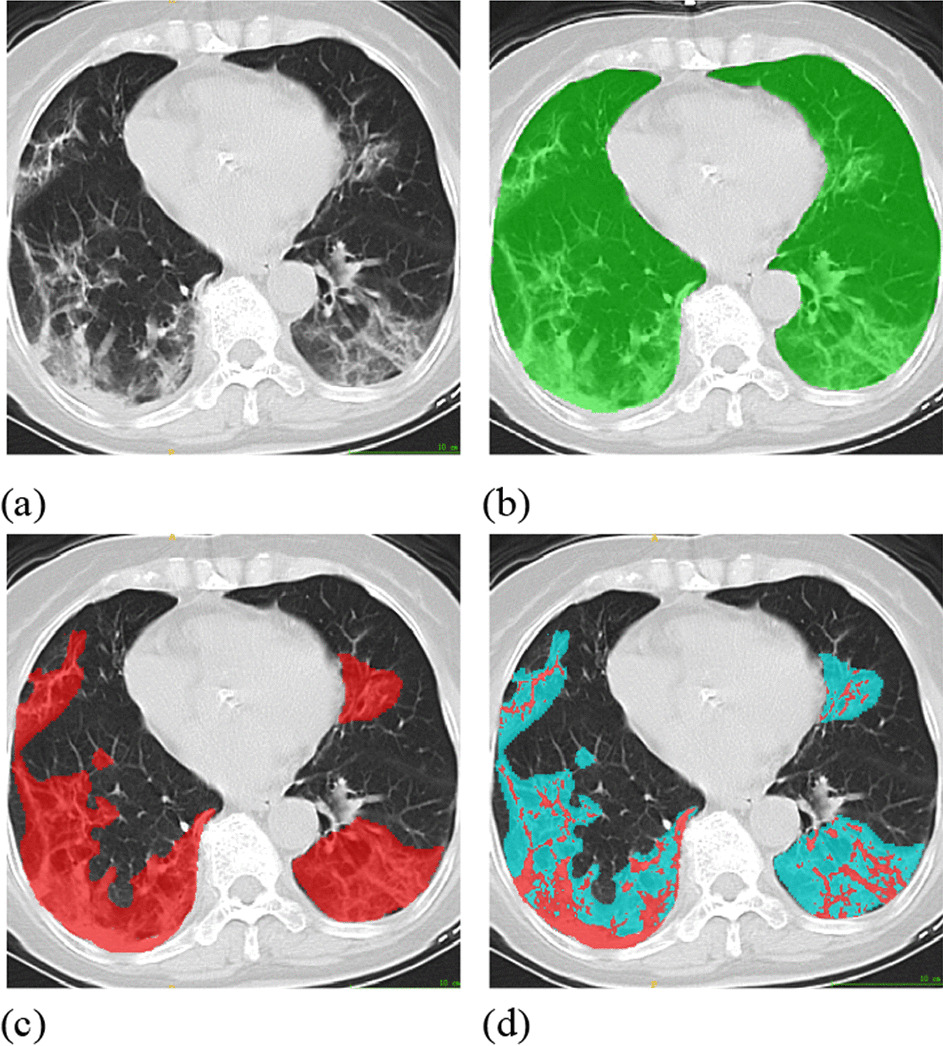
a Original lung CT image; b effective region (or mask of lung); c infected region; d infected region classified by GGO (blue, Hu_[− 750,300]_) and consolidation (light red Hu_[− 300,50]_)
